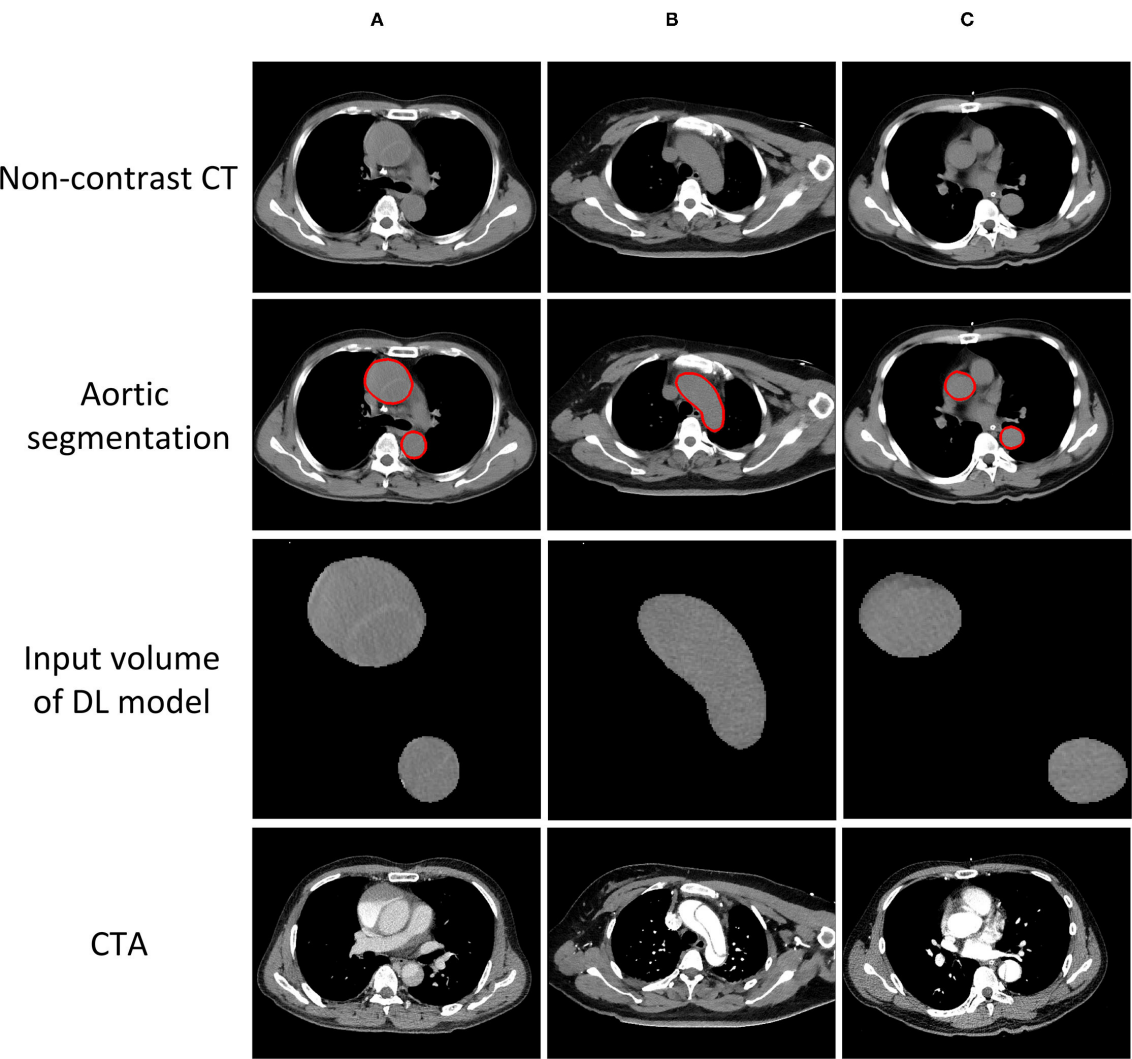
AD cases with CT images. (A) Successfully detected by all the radiologists and the model. (B) Successfully detected by our model, but neglected by one of the radiologists. (C) Neglected by both our model and all the radiologists.
